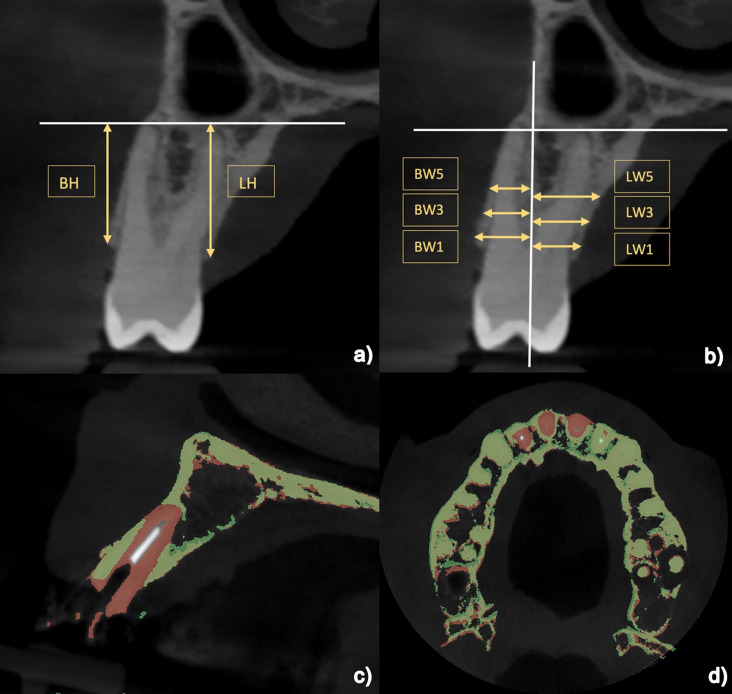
Radiographic measurements on CBCT: a) Vertical references of the buccal and lingual plate height; b) Horizontal references for changes in buccal and lingual width at 1, 3 and 5mm; c) and d) Superimposition of CBCT1 and CBCT2.
